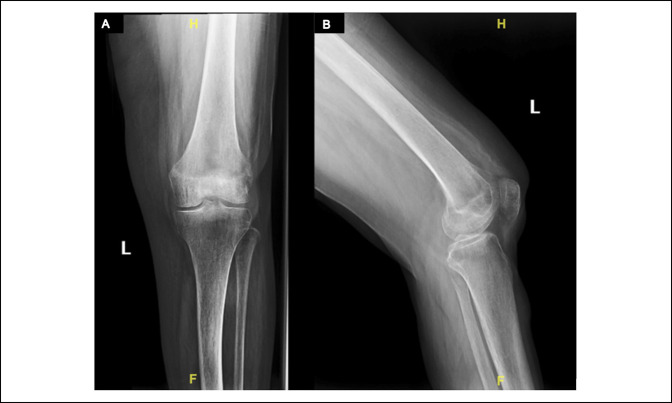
A, Anterior-posterior and (B) lateral radiographs of the left knee demonstrate no acute fracture or dislocation. No evidence of osteolytic or osteoblastic changes about the knee.
